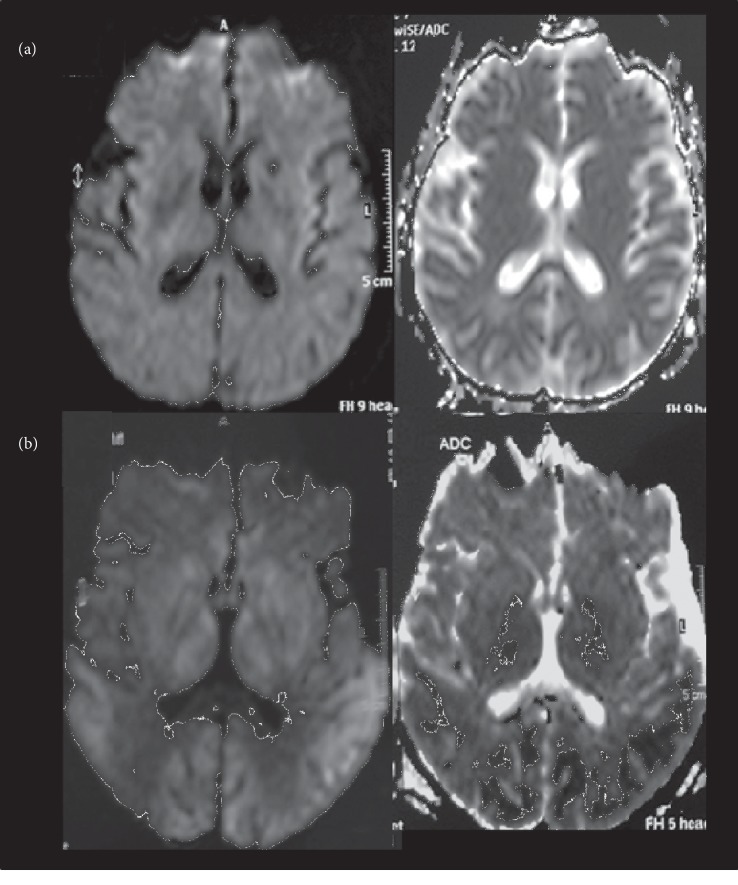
An MRI performed ten days later showed (a) DWI and ADC maps failed to show any abnormality and (b) DWI showed a persistence of bilateral and symmetrical signal abnormalities at the level of temporo-parietal-occipital cortex with reduced ADC map values.
